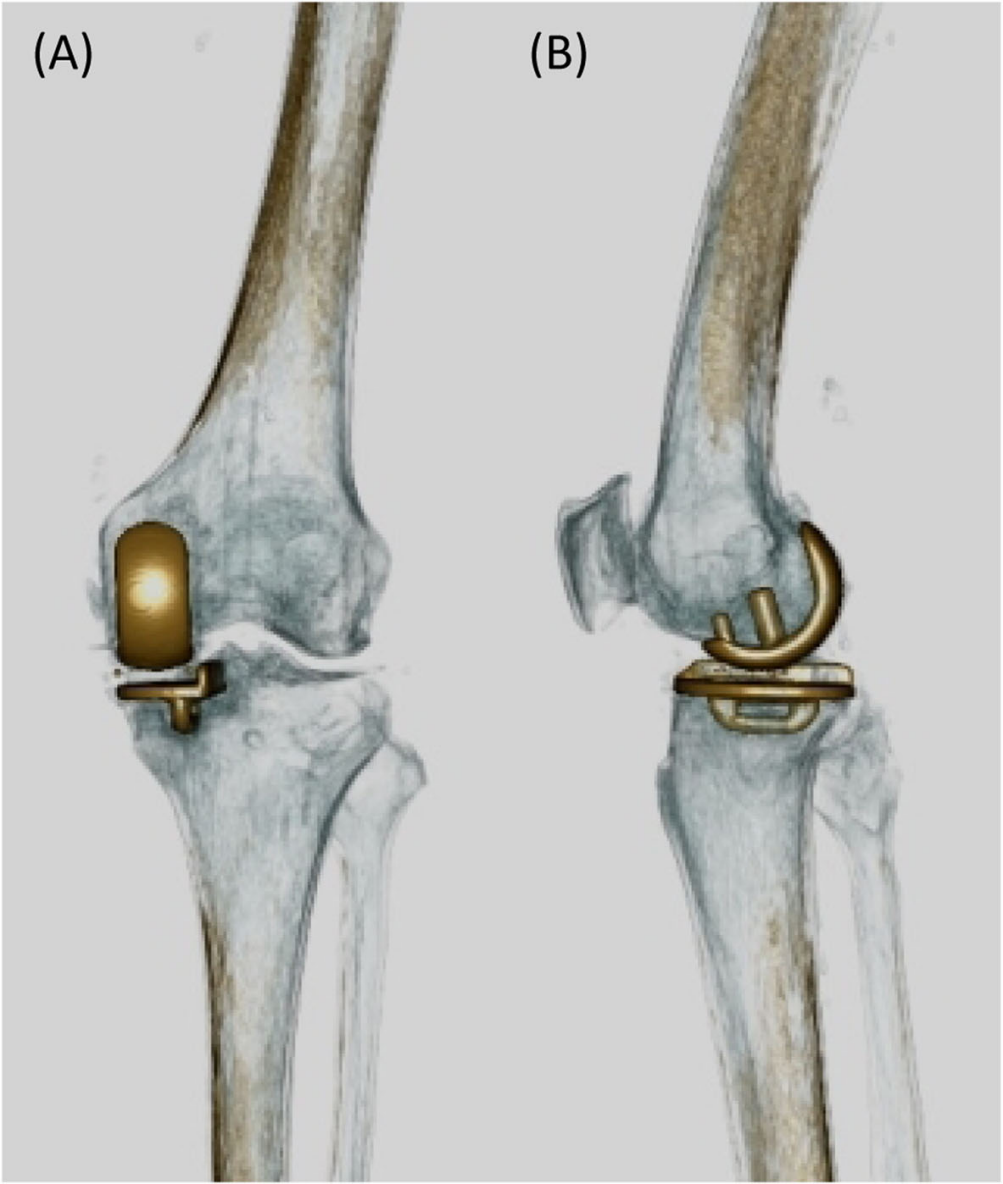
Postoperative 3D CT for assessment of the tibial component. a The image shows evenly distributed bilateral condyles and was selected as the representative image for the measurement film section for the AP view. b The image shows completely overlapping outlines of the lateral and medial femoral condyles and was selected for the measurement of the lateral view
